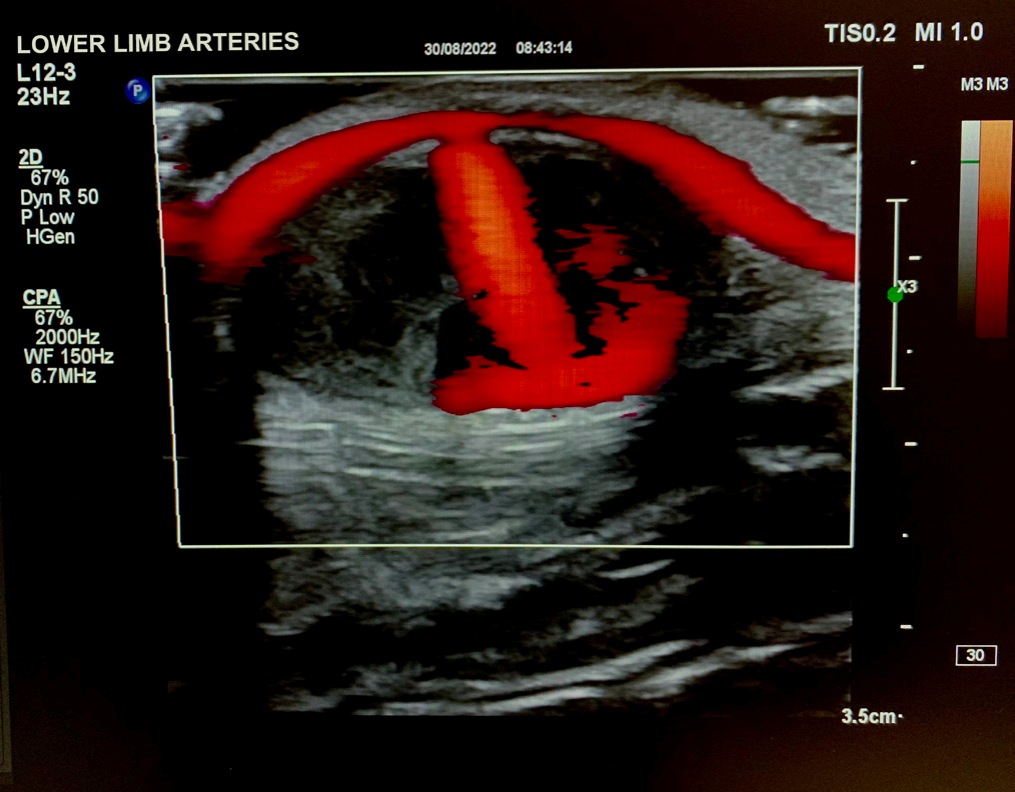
Doppler ultrasonography demonstrating the superficial ulnar artery and arterial flow into the pseudoaneurysm sac with Power Doppler.
